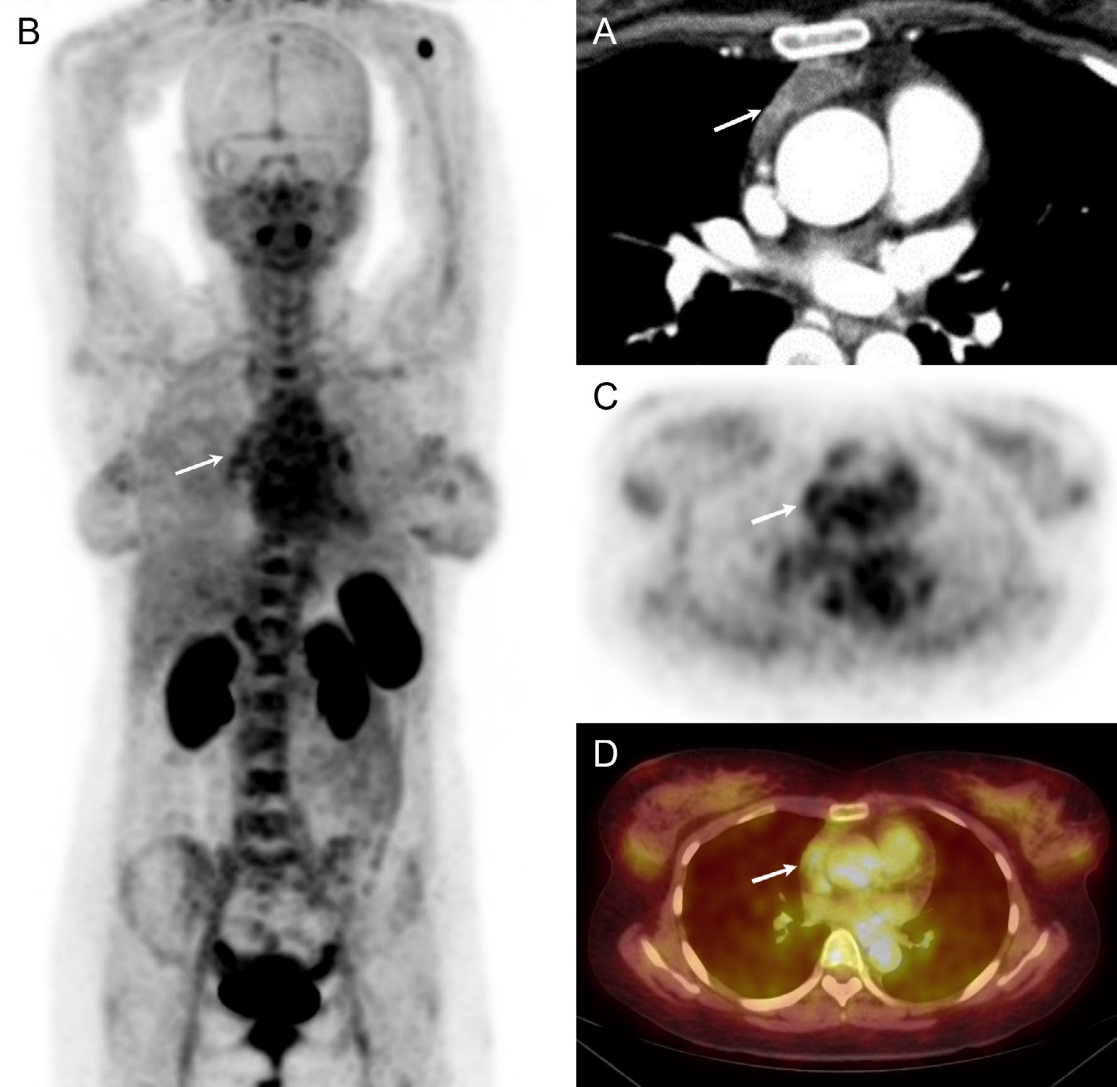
^68^Ga‐Pentixafor PET/CT scans of a thymic cyst MALT lymphoma. Contrast‐enhanced chest CT showing a well‐demarcated mediastinal cyst with mildly enhancing focal thickening of the cystic wall (A). Maximum intensity projections (MIPs) (B), PET axial view (C) and PET/CT fusion (D) of ^68^Ga‐Pentixafor PET/CT showing a thymic cystic MALT lymphoma with mild uptake.
